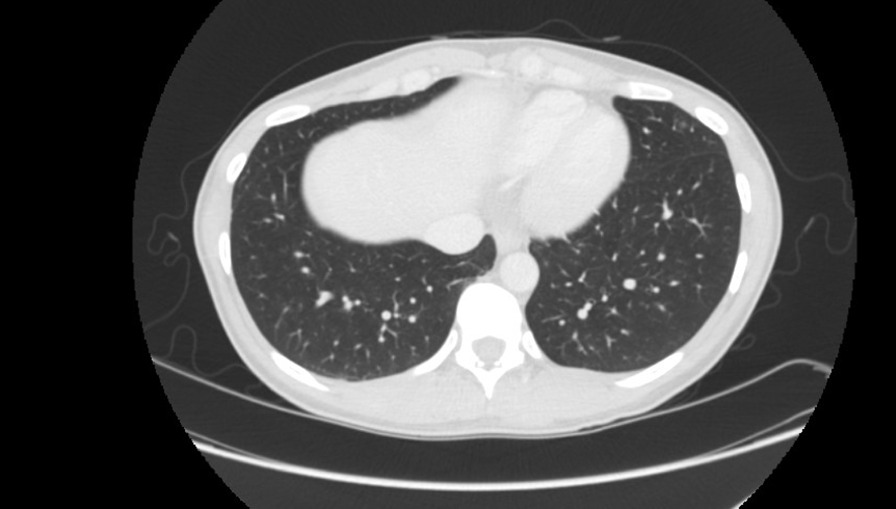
Contrasted abdomen CT scan: A peripheral alveolar, and ground-glass opacities at lung window
